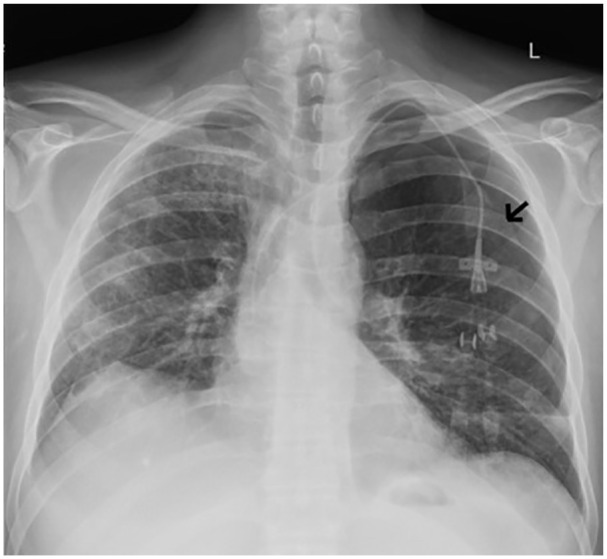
Follow-up chest X-ray performed when the patient was symptomatic (22 hours after transbronchial biopsy). Figure shows the presence of a left-sided apical pneumothorax (black arrow delineates the pleural line).
